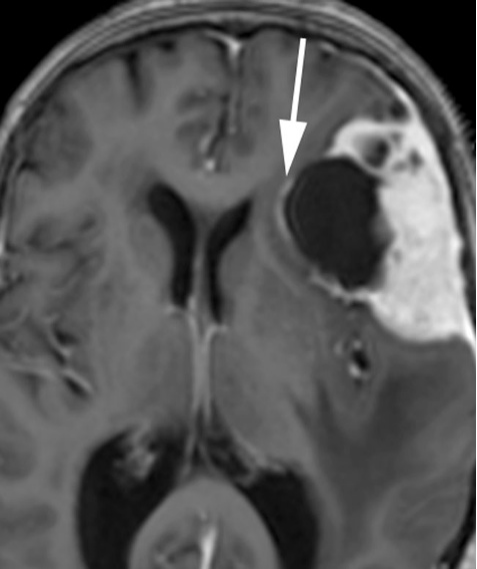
Post-contrast axial T1-weighted sequence in an 80-year-old woman with confusion demonstrates a solid and cystic, extra-axial mass overlying the left frontal lobe with a fine rim of enhancement (*arrow*) around the largest cystic component consistent with a peritumoural cyst. The homogeneously enhancing solid component demonstrated the typical isointensity to grey matter on the T1- and T2-weighted sequences of a meningioma
